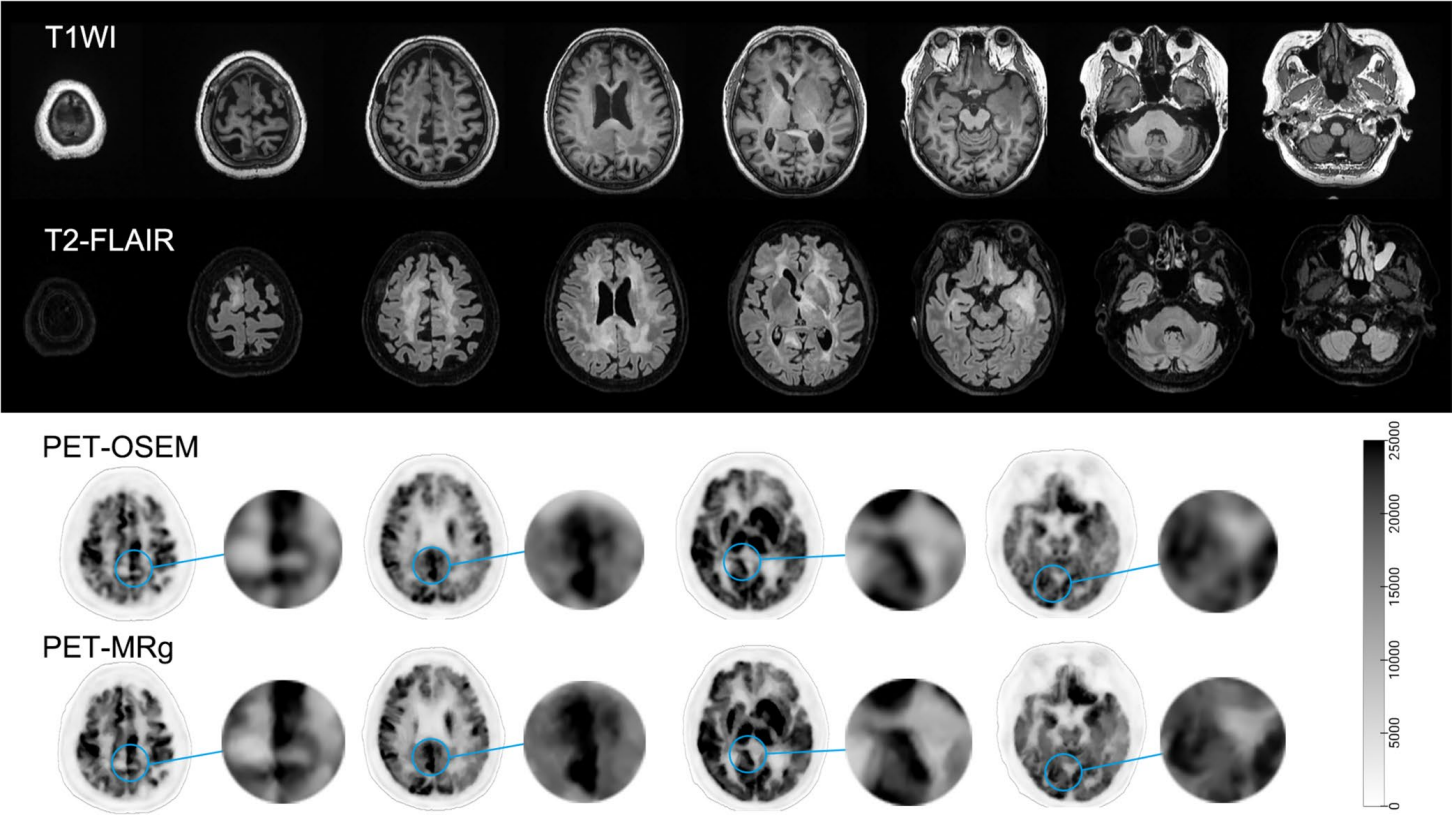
MRg and OSEM PET in a 74‐year‐old female with DLBCL. The MR T1WI (first row) and T2‐FLAIR (second row) images reveal multiple abnormal signal patches in the bilateral cerebral white matter and cortex, predominantly in the left inferior frontal gyrus and left basal ganglia. Visual analysis indicates that the PET‐MRg images have a superior signal‐to‐noise ratio and clearer tissue boundaries compared to the PET‐OSEM images. DLBCL, diffuse large B‐cell lymphoma; FDG, fluorodeoxyglucose; FLAIR, fluid attenuated inversion recovery; MR, magnetic resonance; MRg, magnetic resonance guided PET reconstruction; OSEM, ordered subset expectation maximization; PET, positron emission tomography; T1WI, T1 weighted image.
